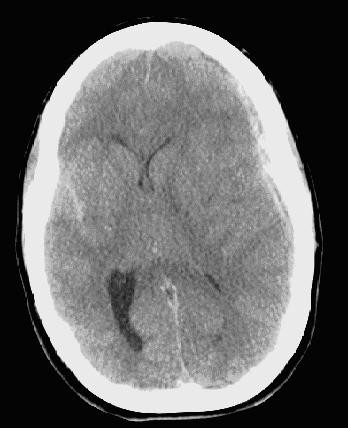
CT scan showing left subdural hematoma with midline shift and right-side subarachnoid hemorrhage.
